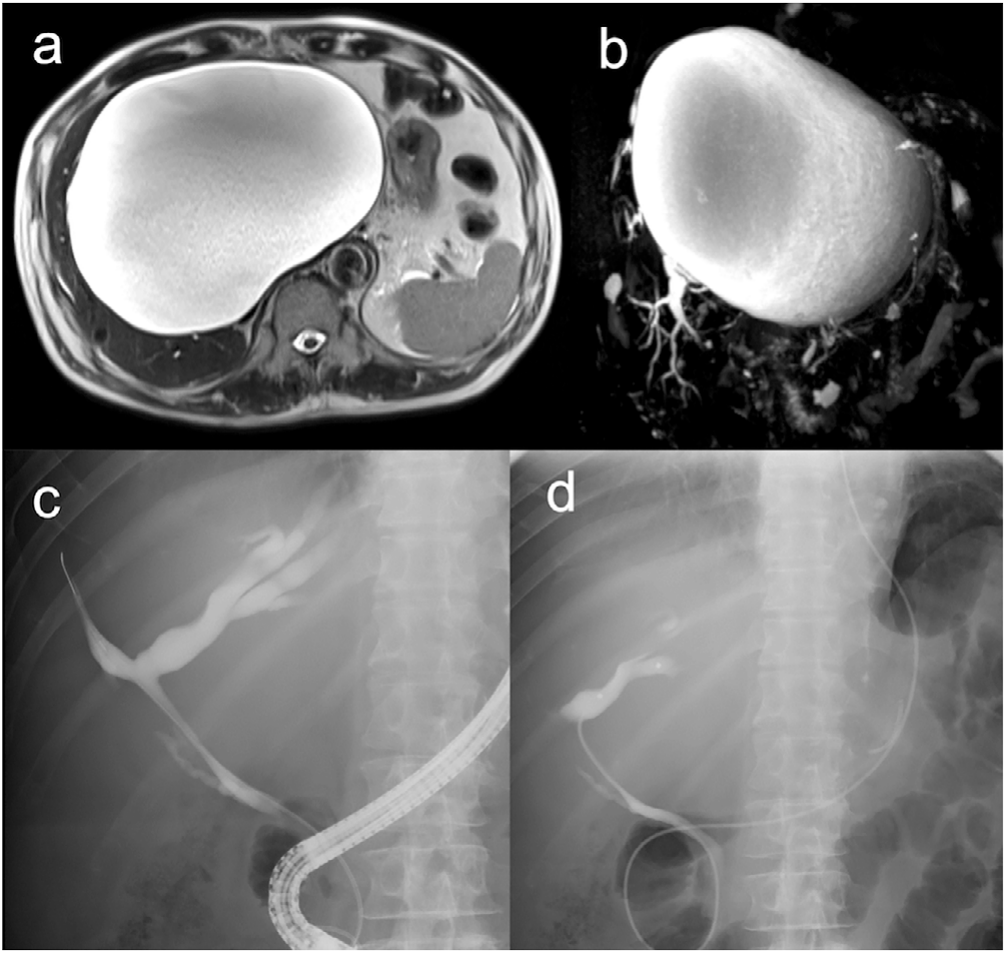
Magnetic resonance and endoscopic retrograde cholangiopancreatography (ERCP) imaging of the giant hepatic cyst and bile ducts. (a) T2‐weighted magnetic resonance imaging (MRI) shows a 20 × 16 cm hepatic cyst mainly in the left lobe, extending into the right lobe. (b) MRCP demonstrates compression of the bile duct by the cyst, with dilation of the intrahepatic bile ducts. (c) ERCP image shows dilatation of the intrahepatic bile ducts due to extrinsic compression by the giant hepatic cyst. (d) ERCP image shows an endoscopic nasobiliary drainage tube in place.
